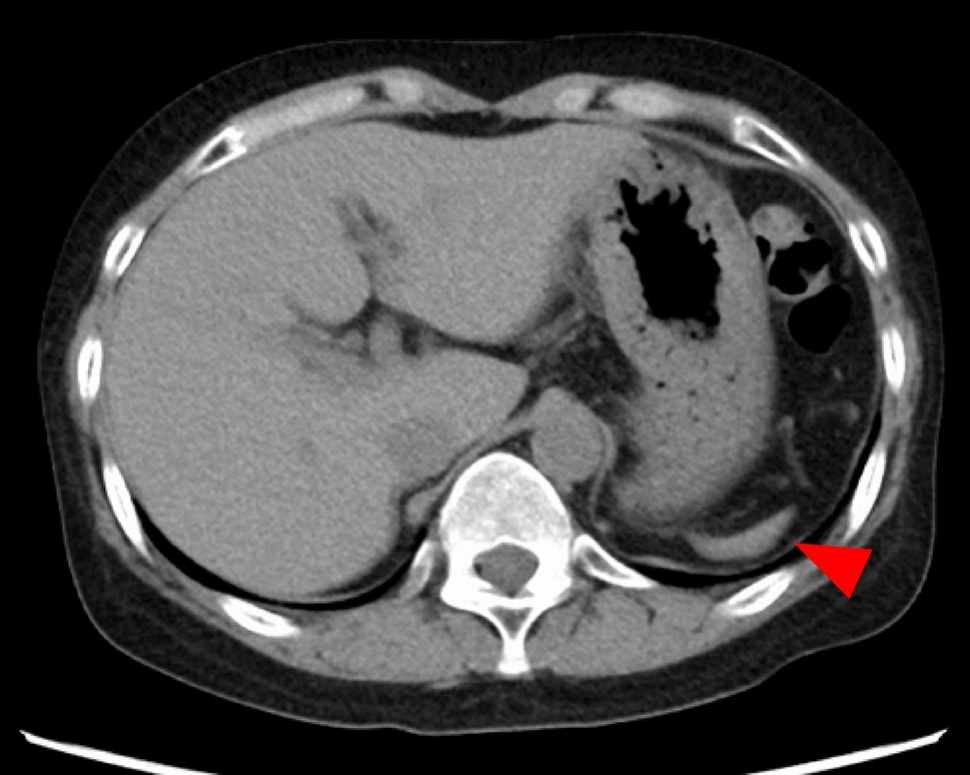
Abdominal CT image of a representative patient demonstrating marked splenic atrophy (red arrow). The measured splenic volume was 5.9 mL. *CT* computed tomography
